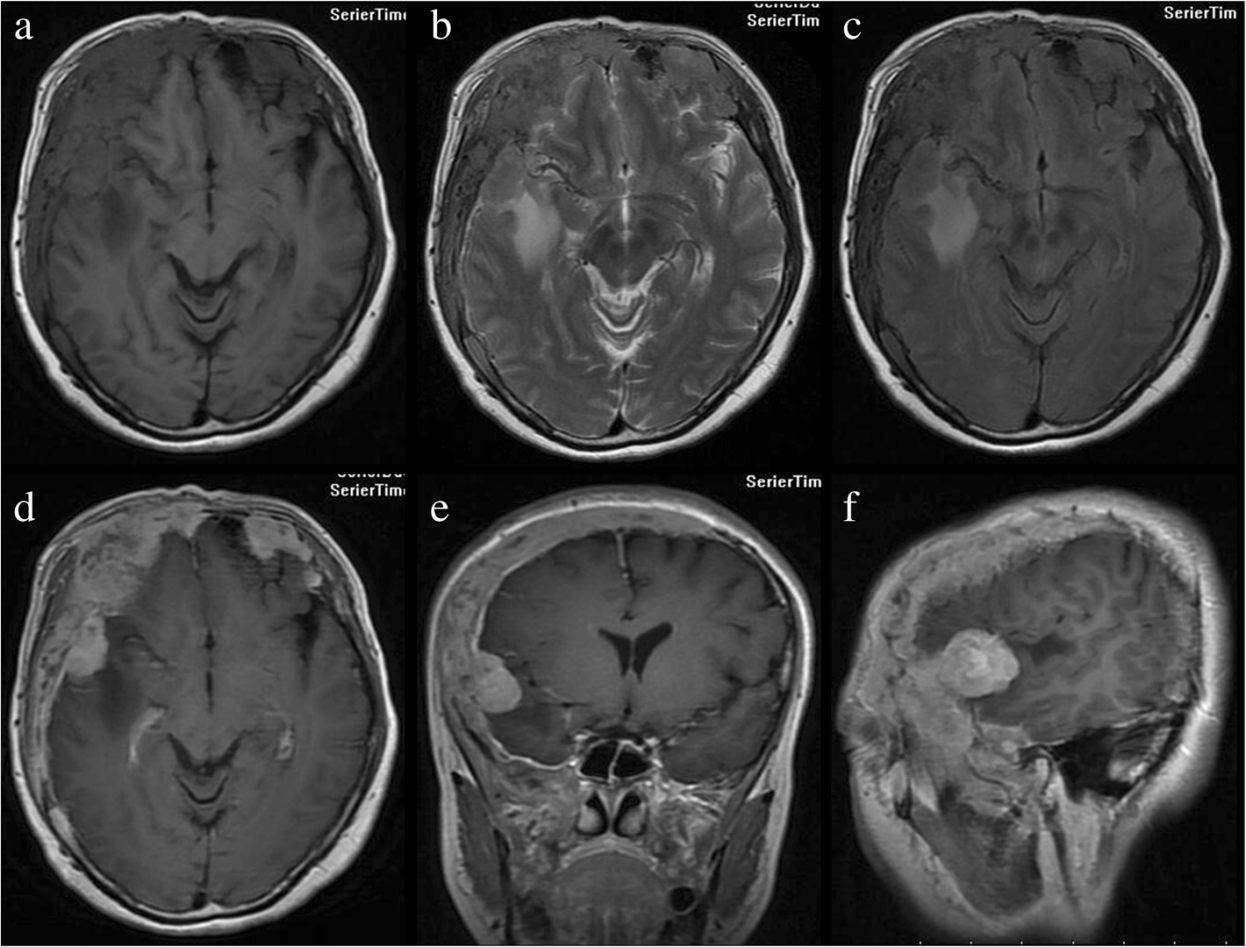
Magnetic resonance imaging revealed that thickening of the bilateral forehead, right superior temporal occipital bone and bilateral upper orbital bone. There were multiple visible flaps and nodules along with slightly longer T1 (a), slightly longer T2 (b), and other FLAIR (c) signals in these areas of bone thickening. An enhanced scan revealed slightly irregular enhancement (d, e, f). Significantly enhanced nodules could be observed on the right side of the temporal and temporal occipital meninges. The largest nodule was at the right side of the temporal lobe, and pressure spread to the adjacent brain parenchyma, around pressure exist the long T1 long T2 edema signal. There was slight compression of the adjacent brain parenchyma and ventricle
